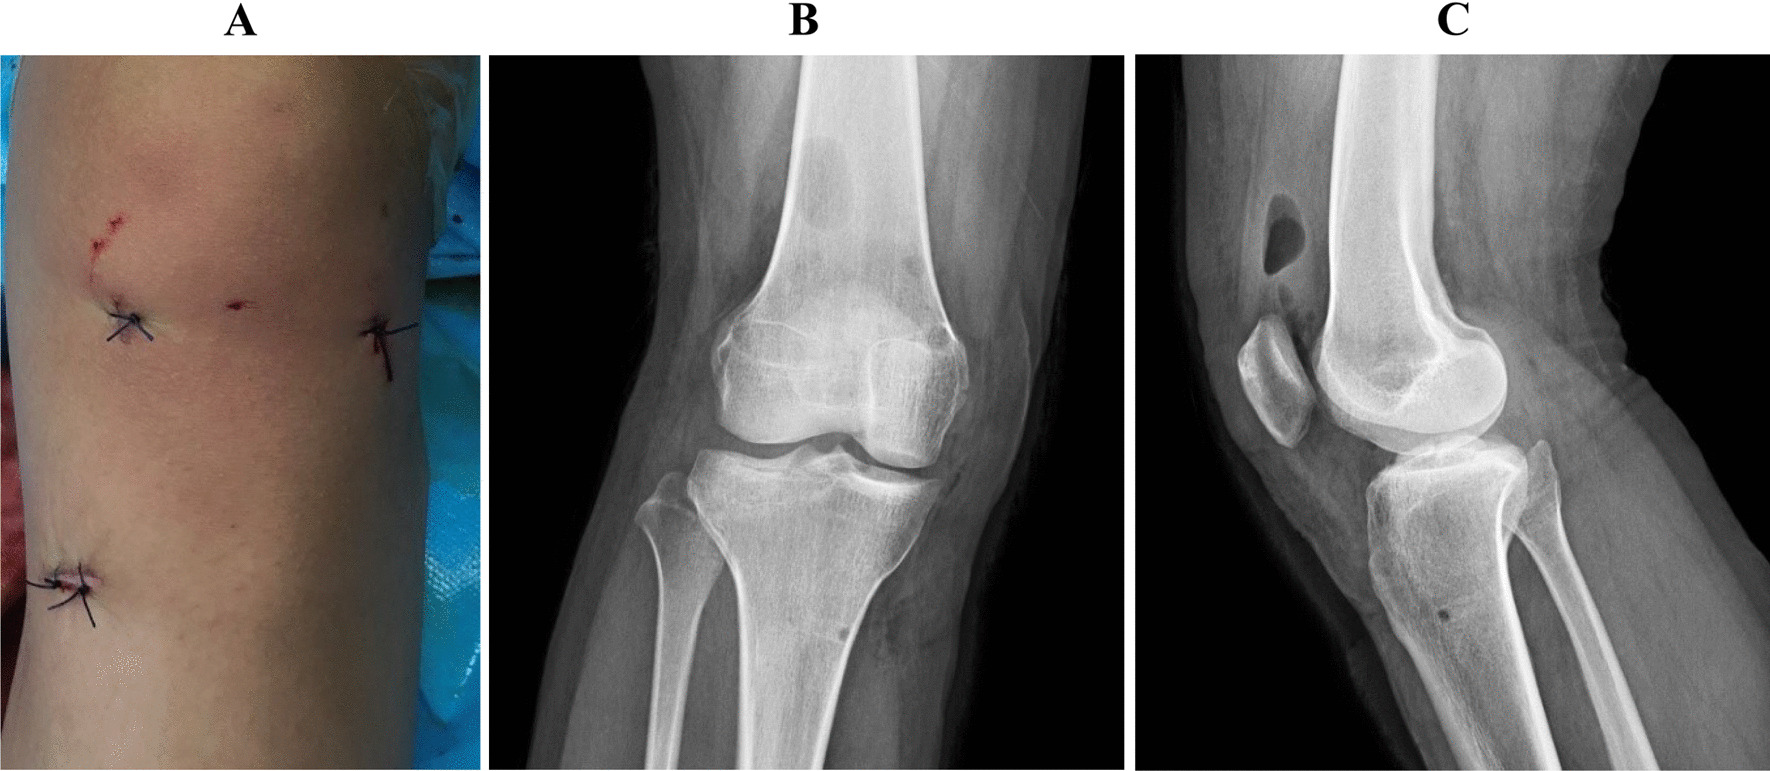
Recovery after operation. A The appearance of the incision after surgery was shown; B, C the results of radiographs were reviewed postoperatively
